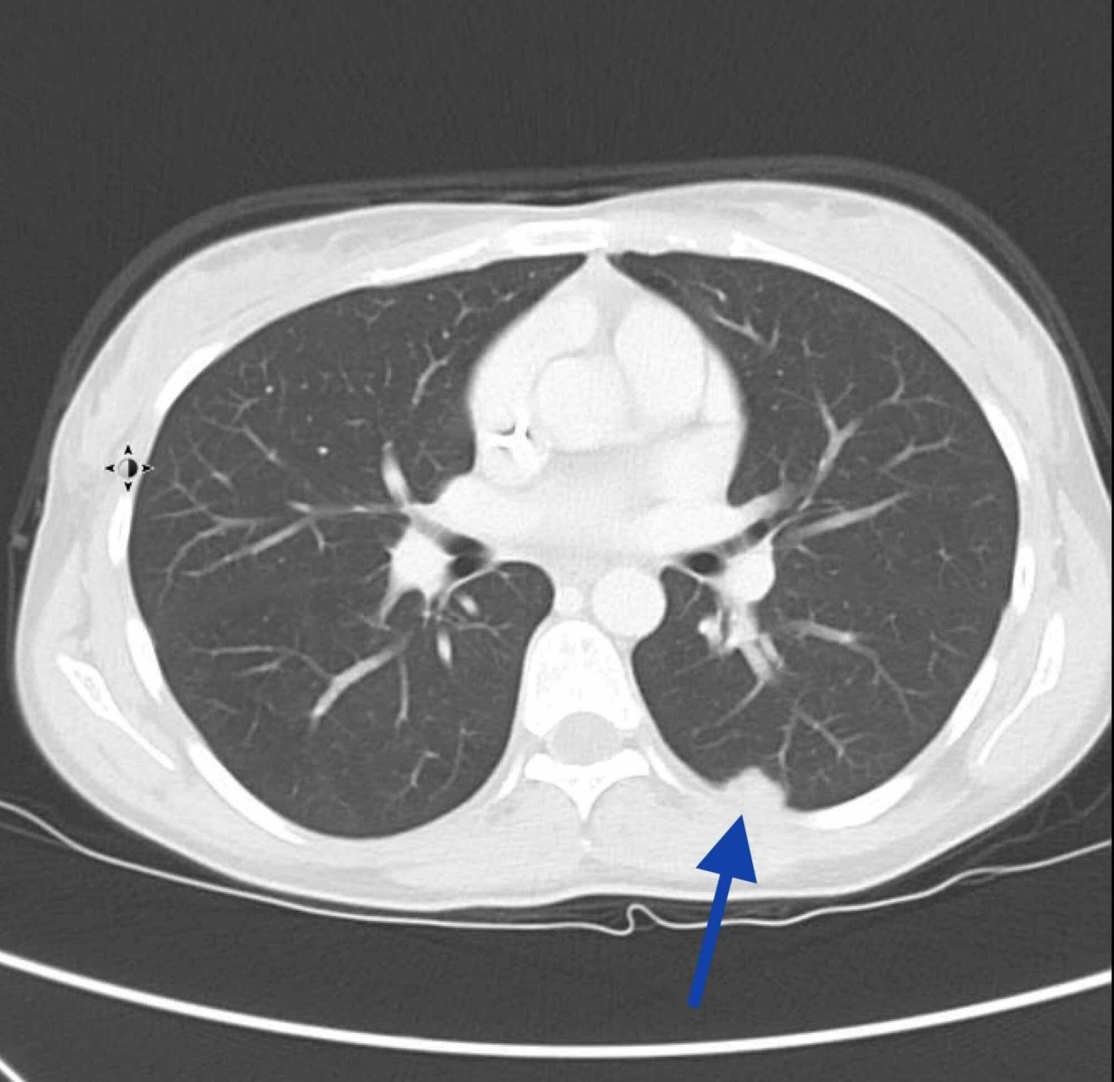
CT scan of axial section showing left lower lobe nodule (blue arrow)
